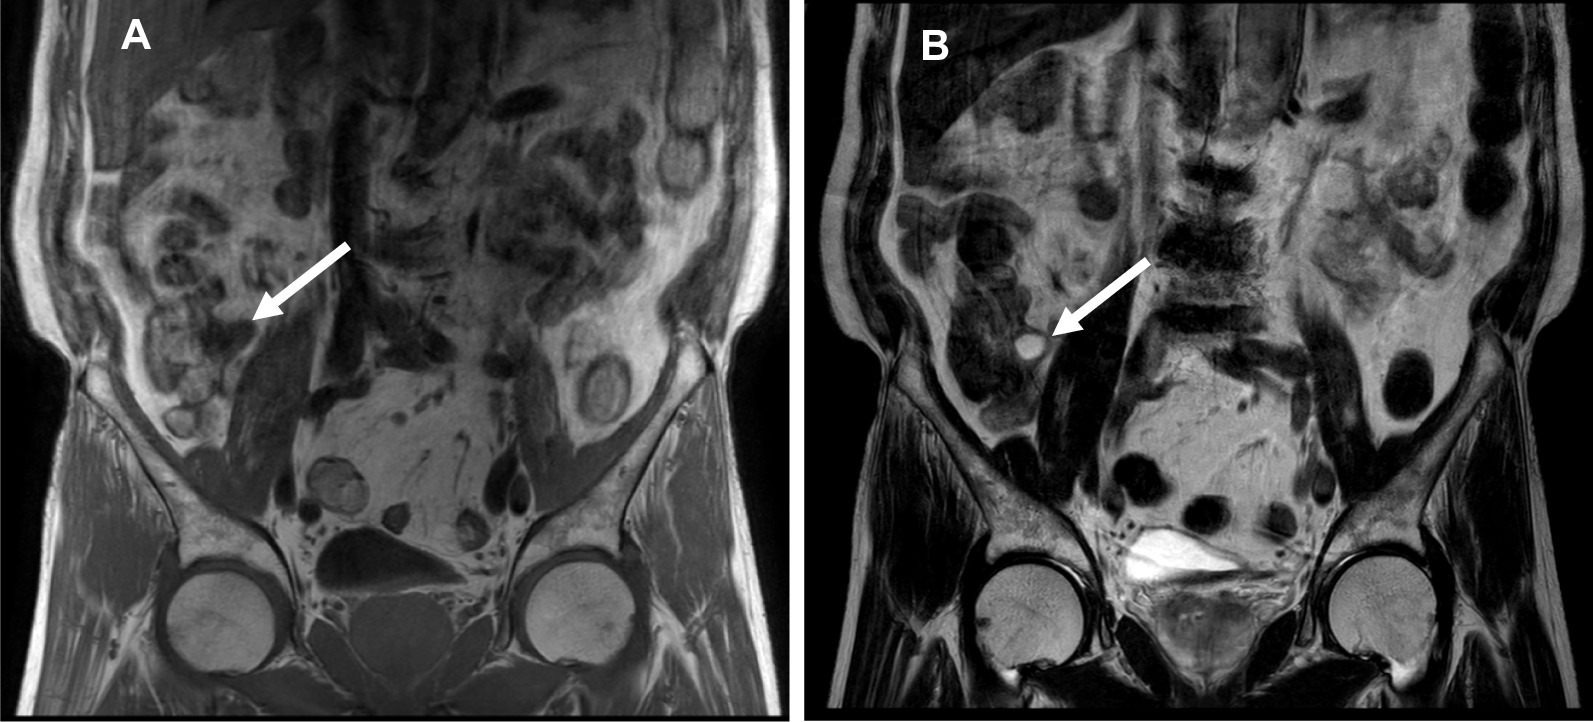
Abdominal magnetic resonance imaging (MRI) (coronal section). MRI revealed a cystic lesion at the appendiceal base with a low intensity on the T1-weighted imaging (a, arrow), and a high intensity on the T2-weighted imaging (b, arrow)
